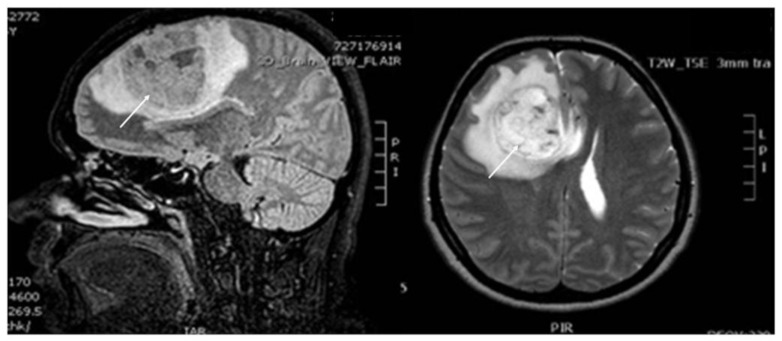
Preoperative brain MRI of the brain at the time of the first diagnosis, 24 months earlier. A heterogenous high signal mass in the right parietal lobe (marked with white arrow), with perifocal edema effacing adjacent sulci, pressure on the right lateral ventricle and shift of the midline to the left including the genu of corpus collusion (Left: FLAIR image, right: T2-weighted image).
